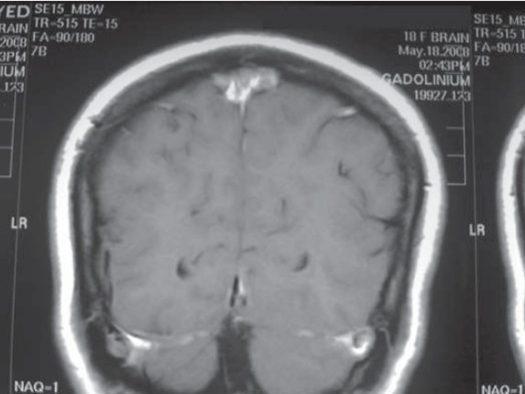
Coronal MRI showing delta sign demonstrating superior sinus thrombosis.
